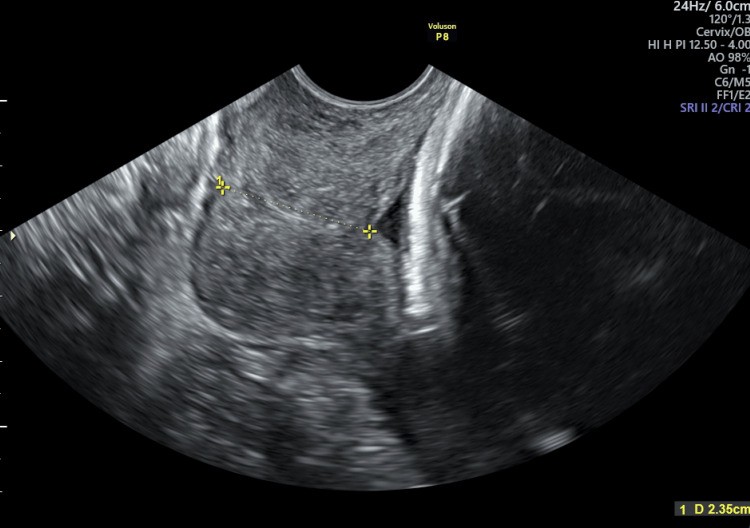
The patient’s midtrimester ultrasound at 30.3 weeks of pregnancy
